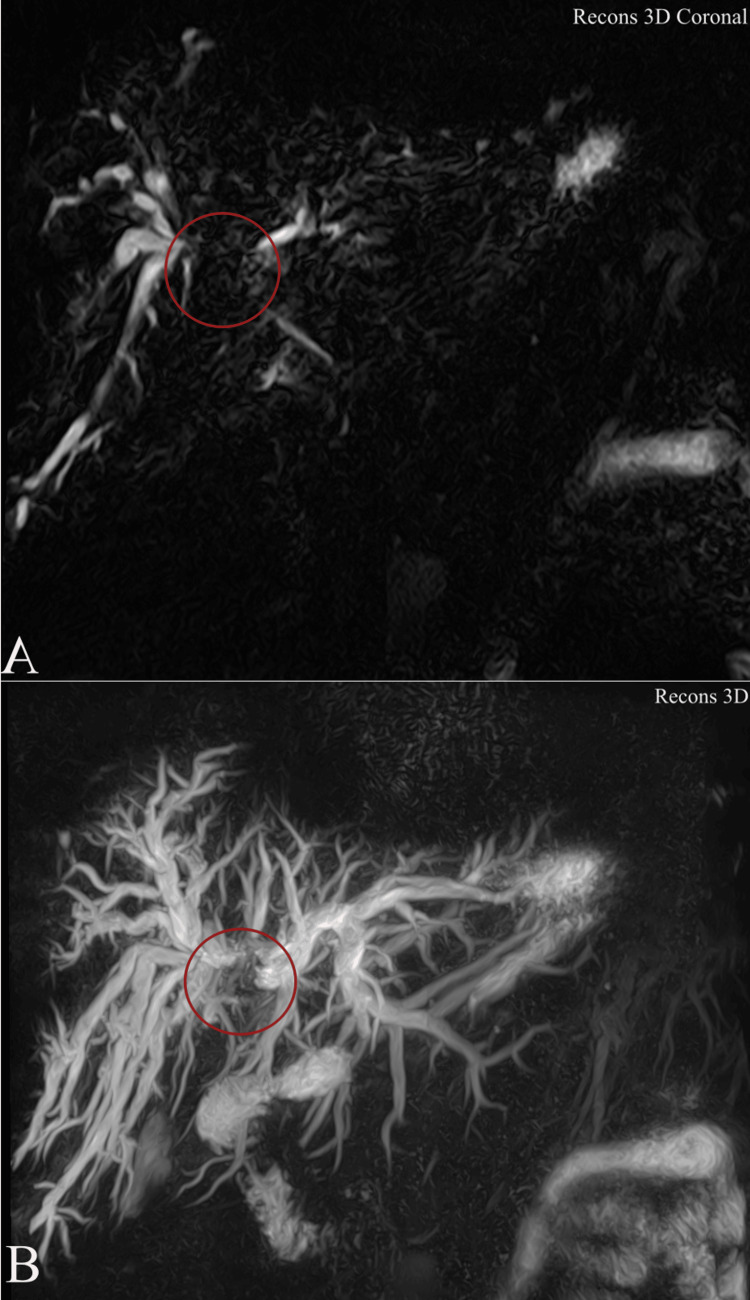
Three-dimensional reconstructions from magnetic resonance cholangiopancreatography in the same patient with Bismuth-Corlette type IIIa perihilar cholangiocarcinoma. (A) Anterior coronal 3D heavily T2-weighted MIP reconstruction showing bilateral intrahepatic biliary dilatation with complete obliteration of the biliary confluence and >1 cm separation of the proximal right and left hepatic ducts (red circle). (B) Slightly oblique inferior 3D view confirming tumor involvement of the proximal right hepatic duct while the left biliary system remains patent. Tumor classification is based on the Bismuth–Corlette classification system as originally described by Bismuth et al. (1992) [3]. MIP: Maximum Intensity Projection
